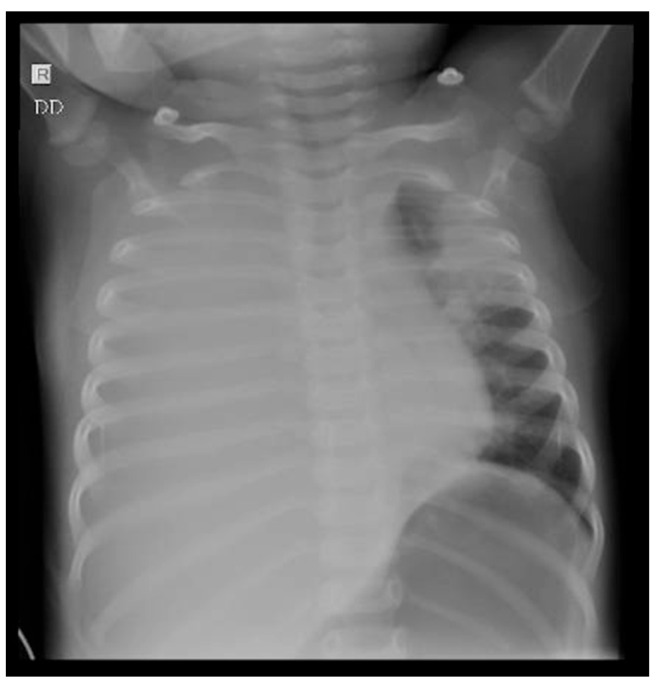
A 15-month-old female infant with hemophagocytic lymphohistiocytosis: pulmonary X-ray showing massive opacity of the right hemithorax, consolidation of the left upper lobe, left perihilar infiltrate.
